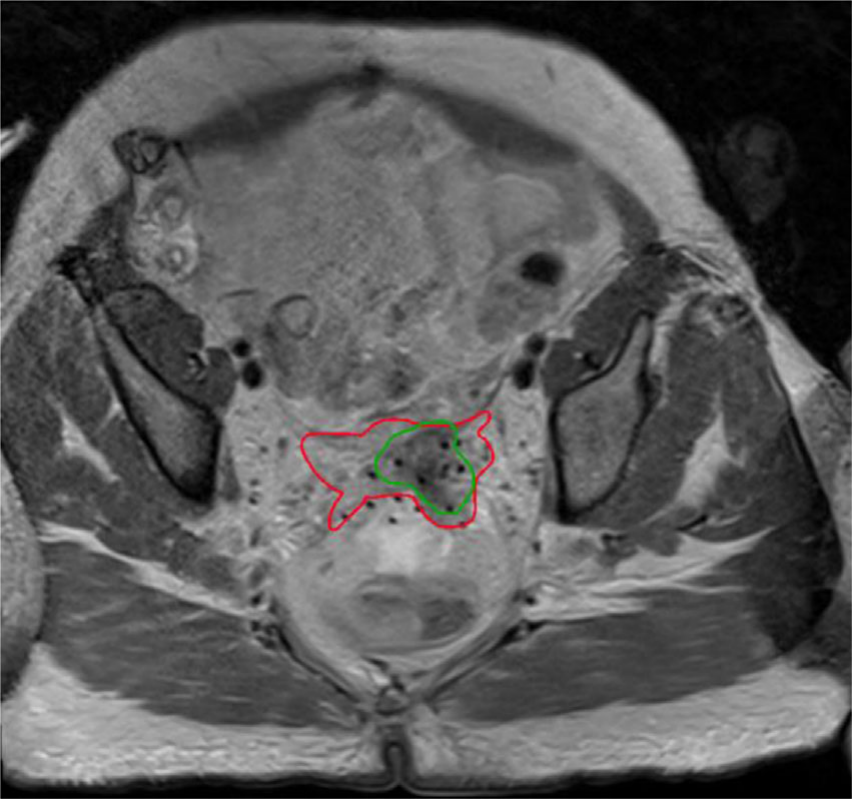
An example of clinical target volume (CTV) (red) and gross tumor volume (GTV) (green) segmentation on an magnetic resonance imaging (MRI) taken at the time of brachytherapy
